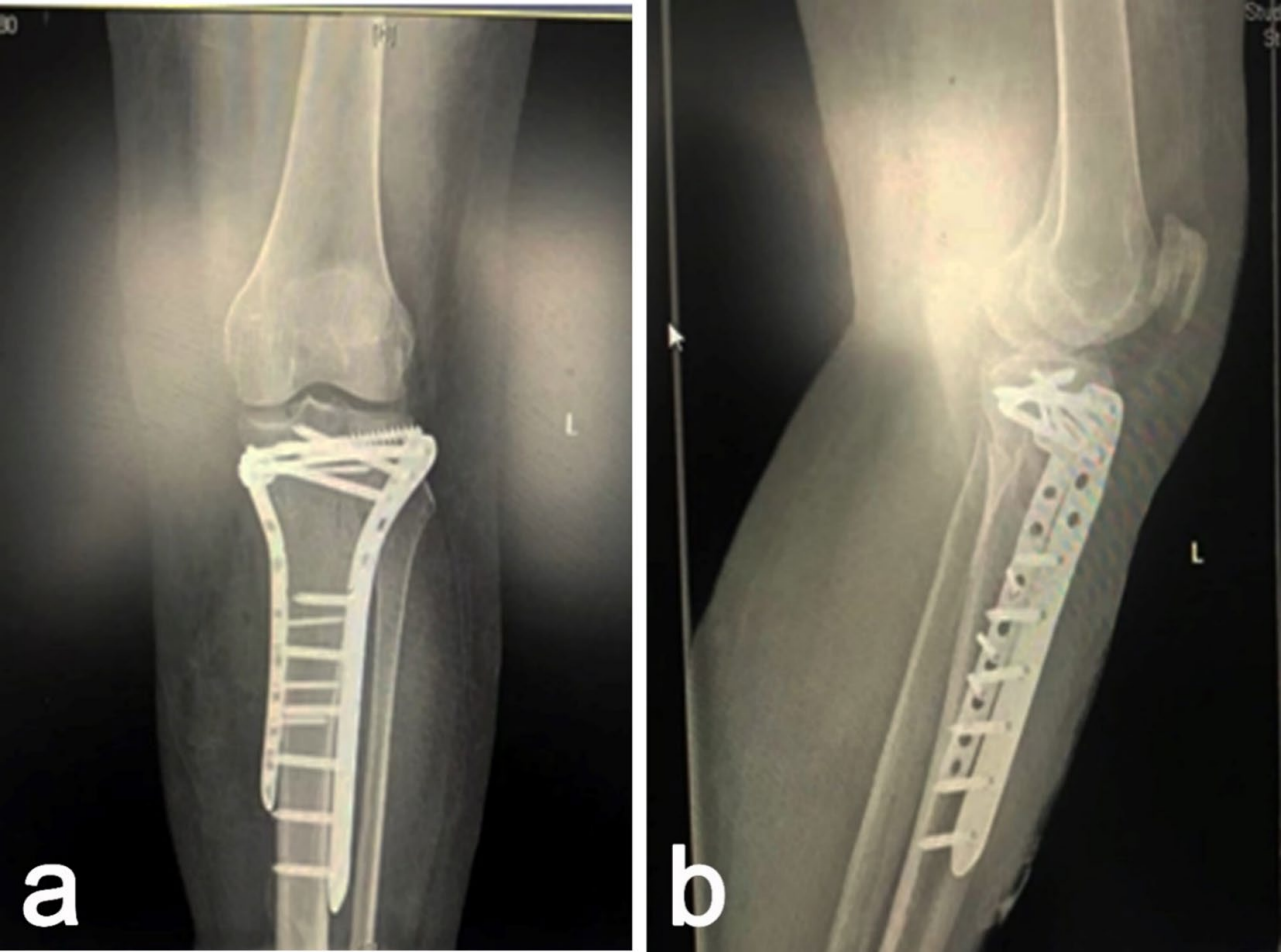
Postoperative X-rays showed that the fracture was well fixed.
